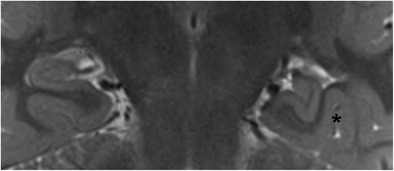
Isolated IHI. Coronal 3-T T2-weighted image in a 23-year-old patient with epilepsy shows a normal hippocampus on the right compared to an incompletely inverted hippocampus with an abnormal rounded or pyramidal shape on the left. The left collateral sulcus (asterisk) has a more vertical orientation and is found lateral of the hippocampal body
